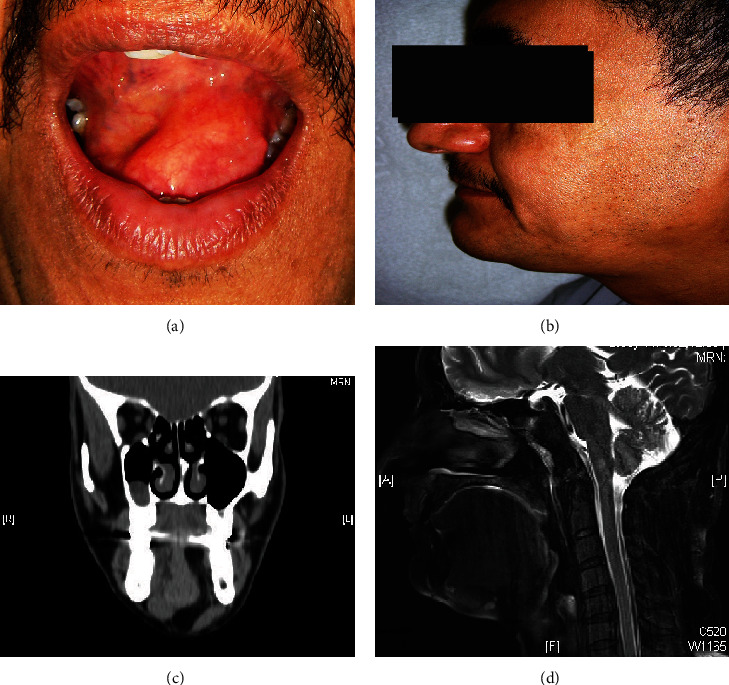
(a) Intraoral soft tissue mass in the floor of the mouth from the left ventral tongue surface extending beyond the midline to the right side. (b) Mild submental swelling as a result of an intraoral mass herniation beyond the mylohyoid muscle. (c) Coronal CT section at the level of the first molar in soft tissue window showing a markedly low density and clearly defined margin irregular mass within the left genioglossus muscle extending from ventral tongue to skin overlying the mylohyoid muscle. The density is homogenous and similar to adjacent subcutaneous fat. (d) Midsagittal MRI section in fat suppression showing the hypointense density mass.
